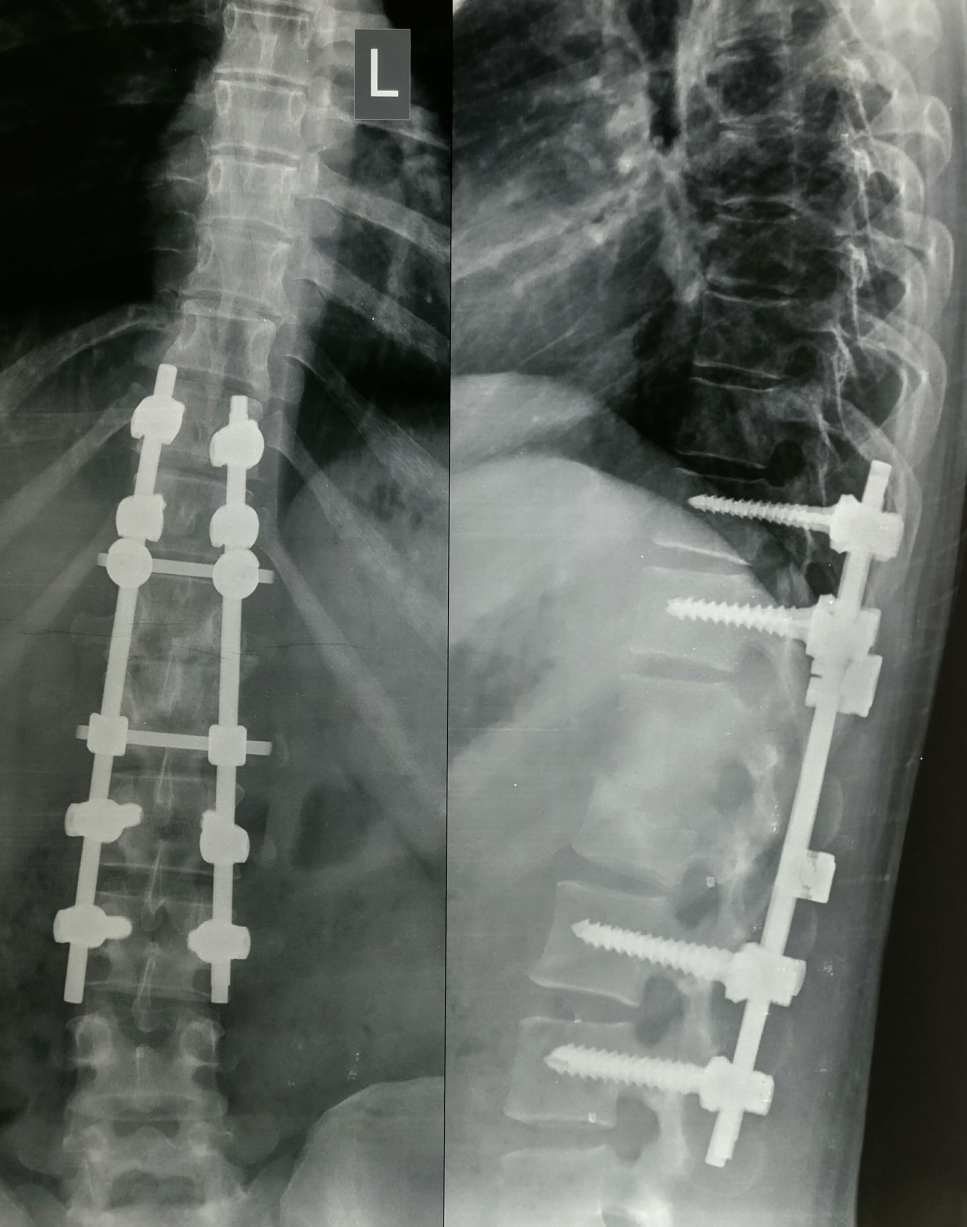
Follow-up radiograph at 22 months Shows fusion of the affected vertebrae and healing of the lesion with sclerotic bone with maintenance of the correction.
